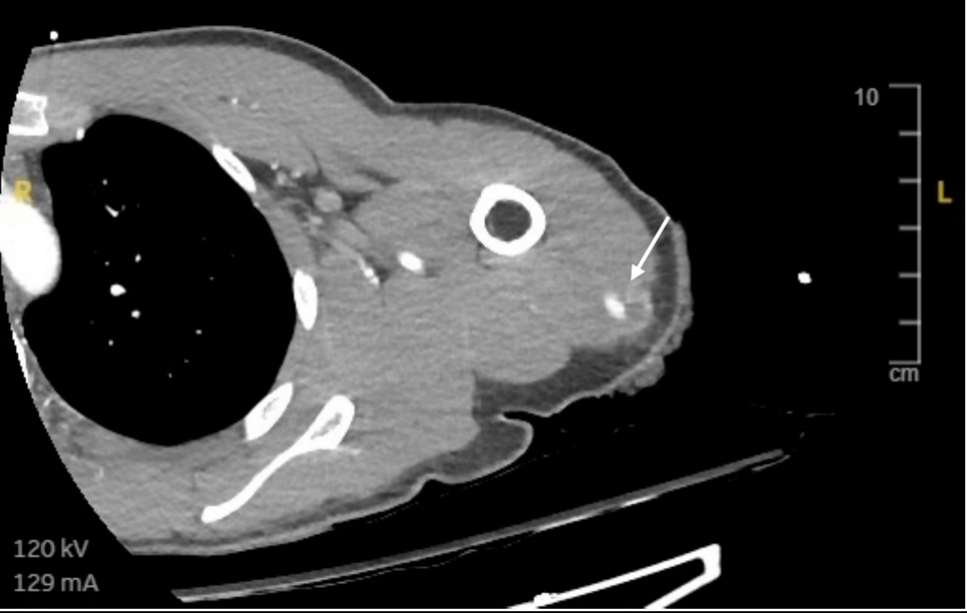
22-year-old male presenting to the emergency department after being stabbed in the left arm. CT scan demonstrated a left upper arm posterior compartment haematoma with contrast extravasation (arrow). The patient was successfully managed conservatively
